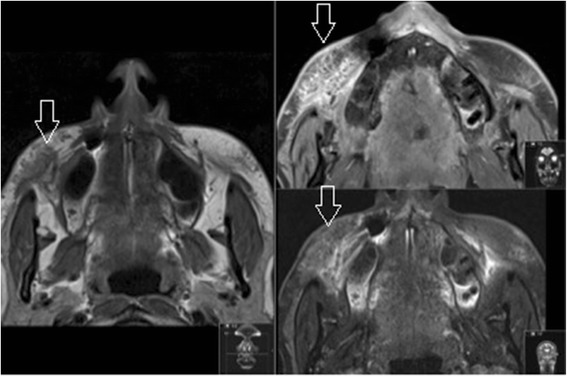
Silicone dermal filler in the right infraorbital triangle (*open arrow*) as seen on axial MRI (T1, T1FS with gadolinium, T2FS). Note how the free silicone oil drops behave like fat on MRI, including a intermediate to low signal on fat suppressed sequences
